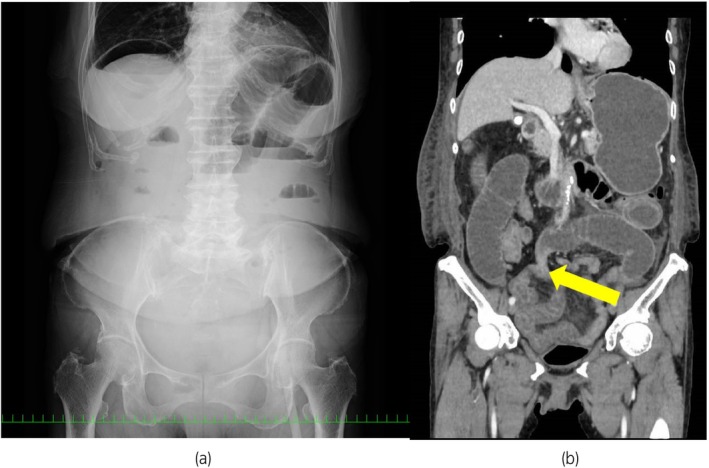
(a) Abdominal X‐ray on the 4th postoperative day showed bowel distension. (b) CT scan on the 11th postoperative day showed distended small bowel and compressed bowel (arrow).
